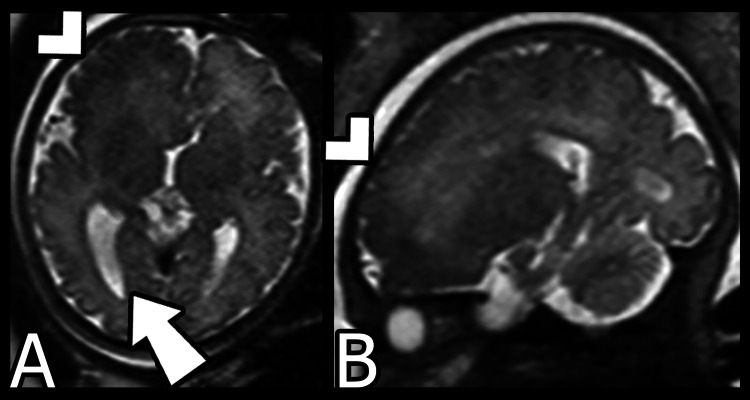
Fetal MRI at 36 weeks’ gestation A) Axial T2-weighted, B) Sagittal T2-weighted. The previously focal abnormality has evolved into a more diffusely dysplastic appearance of the right frontal lobe. The arrowheads indicate an area of cortical thickening and irregular gyration which suggest diffuse cortical malformation. The arrow demonstrates a distinctly enlarged right occipital horn.
